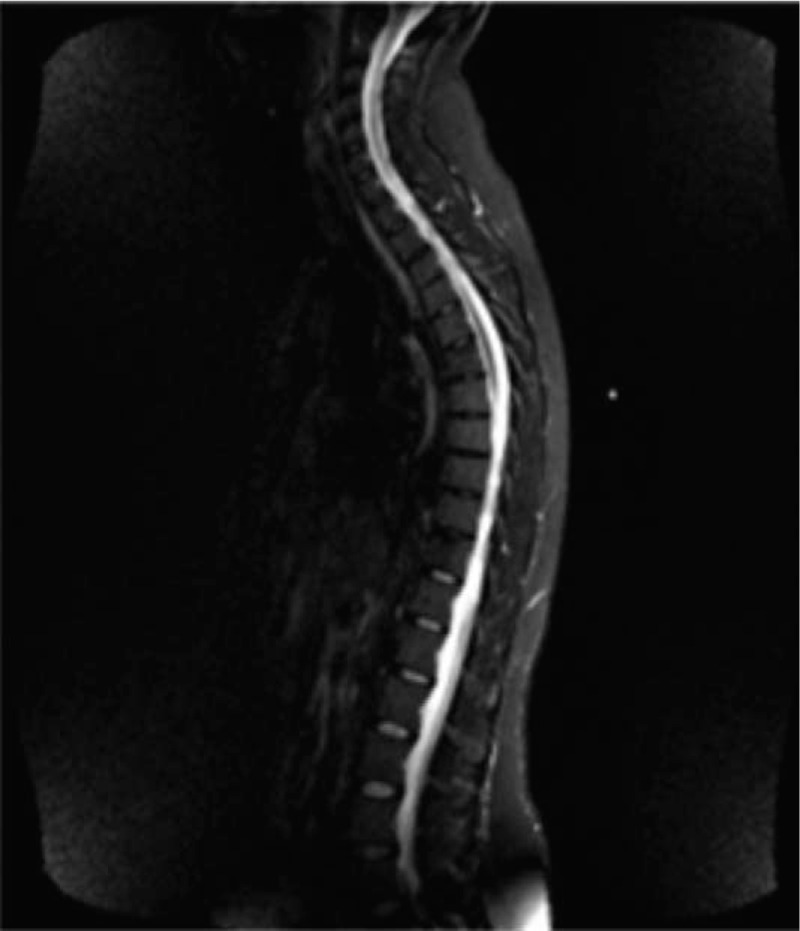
MRI after 6-mo of chemotherapy (February 2007). MRI = magnetic resonance imaging.
